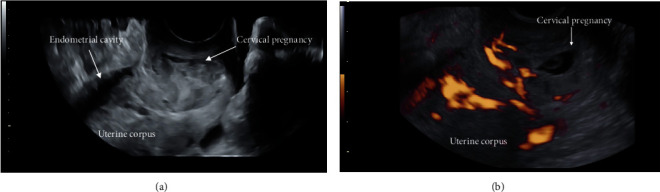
Cervical ectopic pregnancy in (a) 2D and (b) 3D transvaginal ultrasound.
